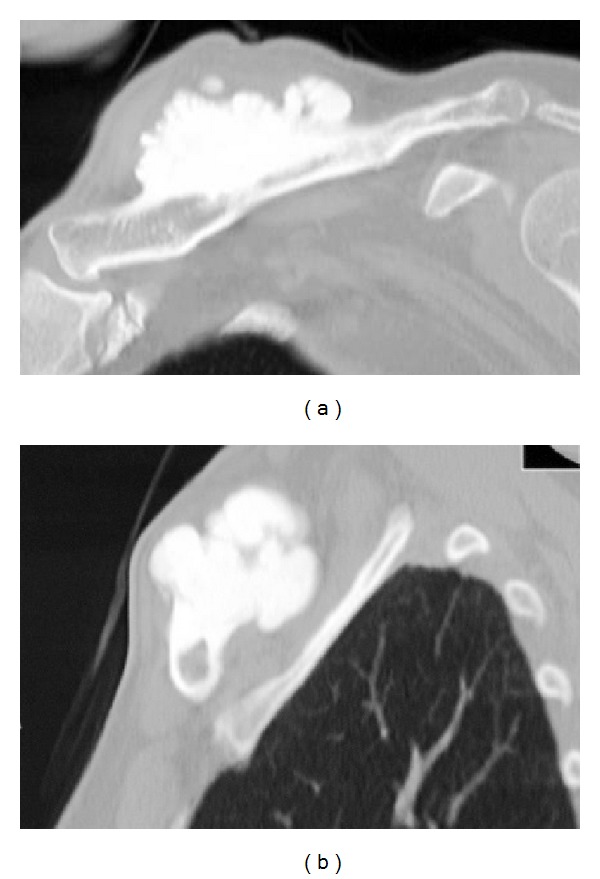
Computed tomography scan reveals that the heavily ossified mass is attached to the cortex and has no areas of lucency. The cortex underlying the osteoma is intact. (a) Coronal image of the left clavicle; (b) sagittal image of the left clavicle.
